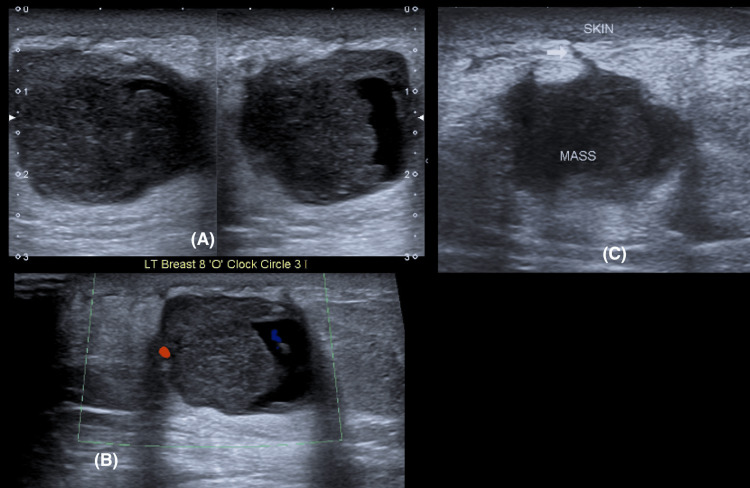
Ultrasound shows a round predominantly solid mass with crescenting cystic spaces (A) and marked posterior acoustic enhancement (B). Linear extension to the skin is also noted (C).
